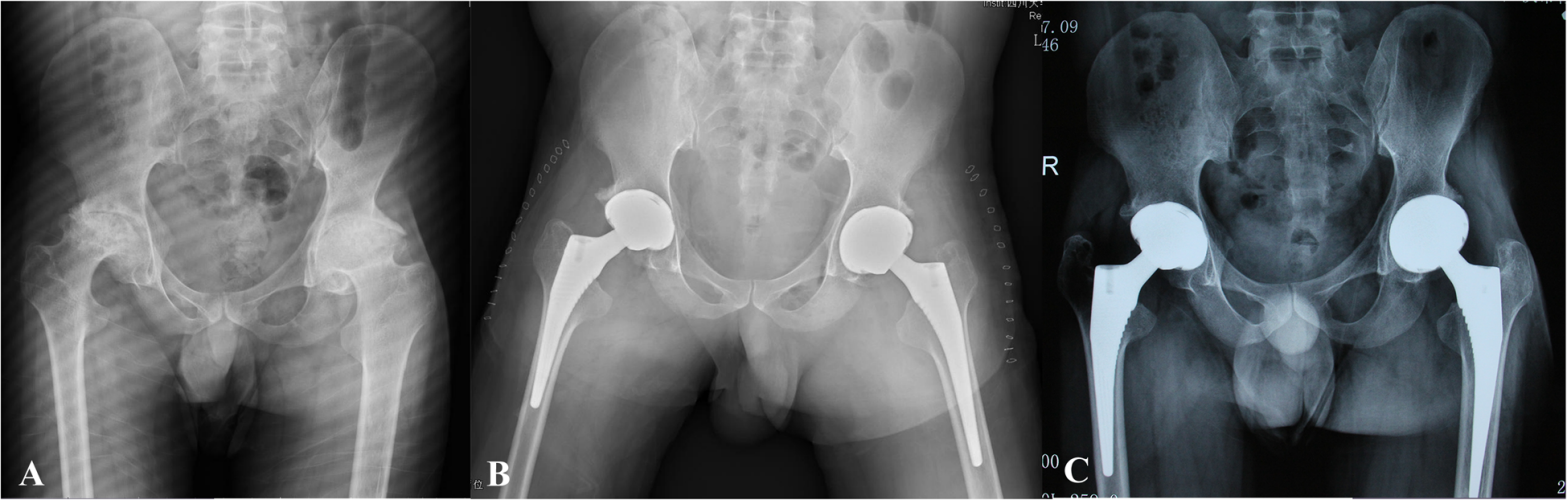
Preoperative and postoperative X-ray of a patient seeking for bilateral THA because of haemophilia A. a Preoperative anteroposterior (AP) view of pelvis; b Posoperative AP view of pelvis; c One year follow-up AP view of pelvis (No progressive radiolucent line > 2 mm in width)
